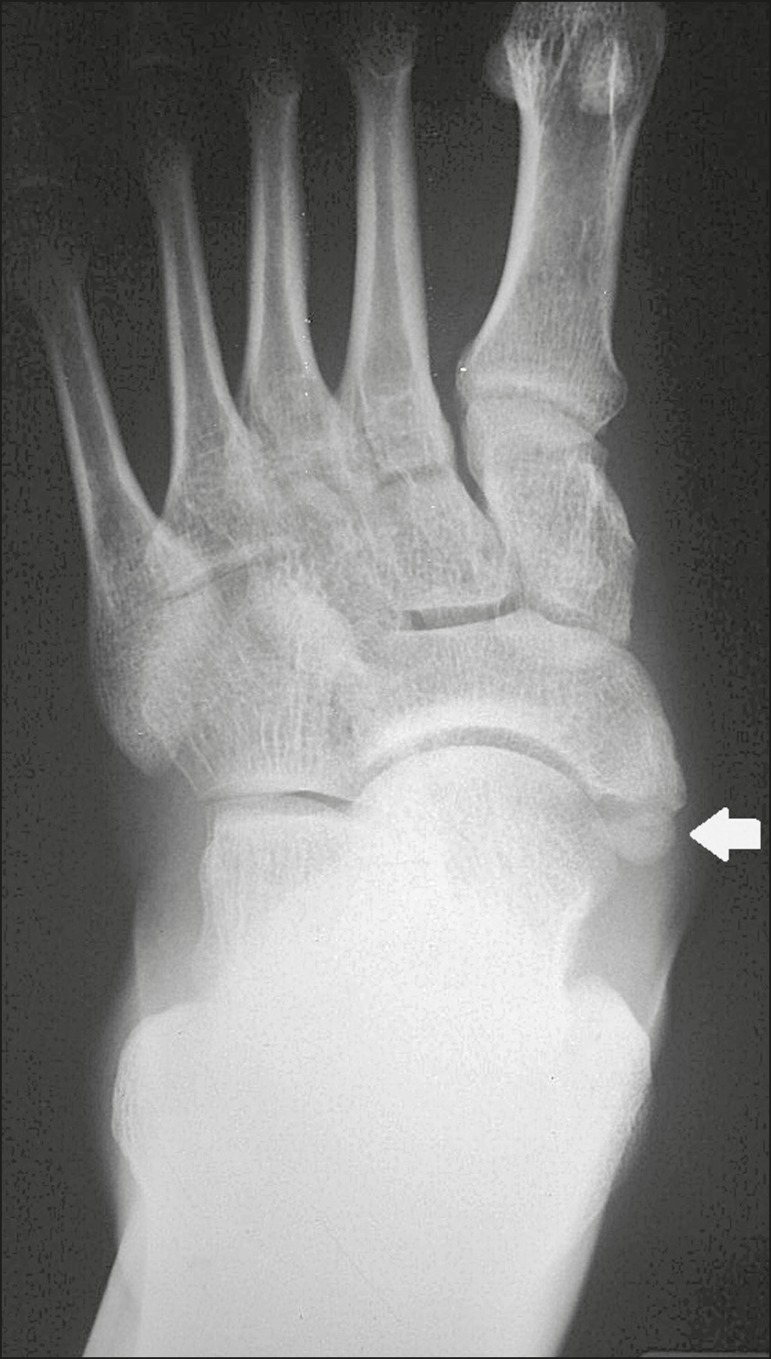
Anteroposterior X-ray of the left foot showing a triangular ossicle
